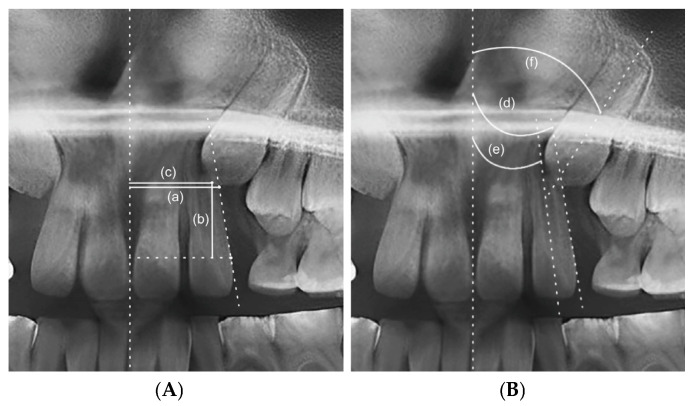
Panoramic radiographs showing: (A) Linear measurements (solid lines): (a) 2DBlock-ML (2DHorz), (b) 3Cusp-2DCEJ (3Vert), and (c) 3Cusp-ML (3Horz); (B) Angular measurements (solid lines): (d) 2D^ML, (e) 2^ML, and (f) 3^ML. Detailed descriptions of all measurements are provided in Table 2.
